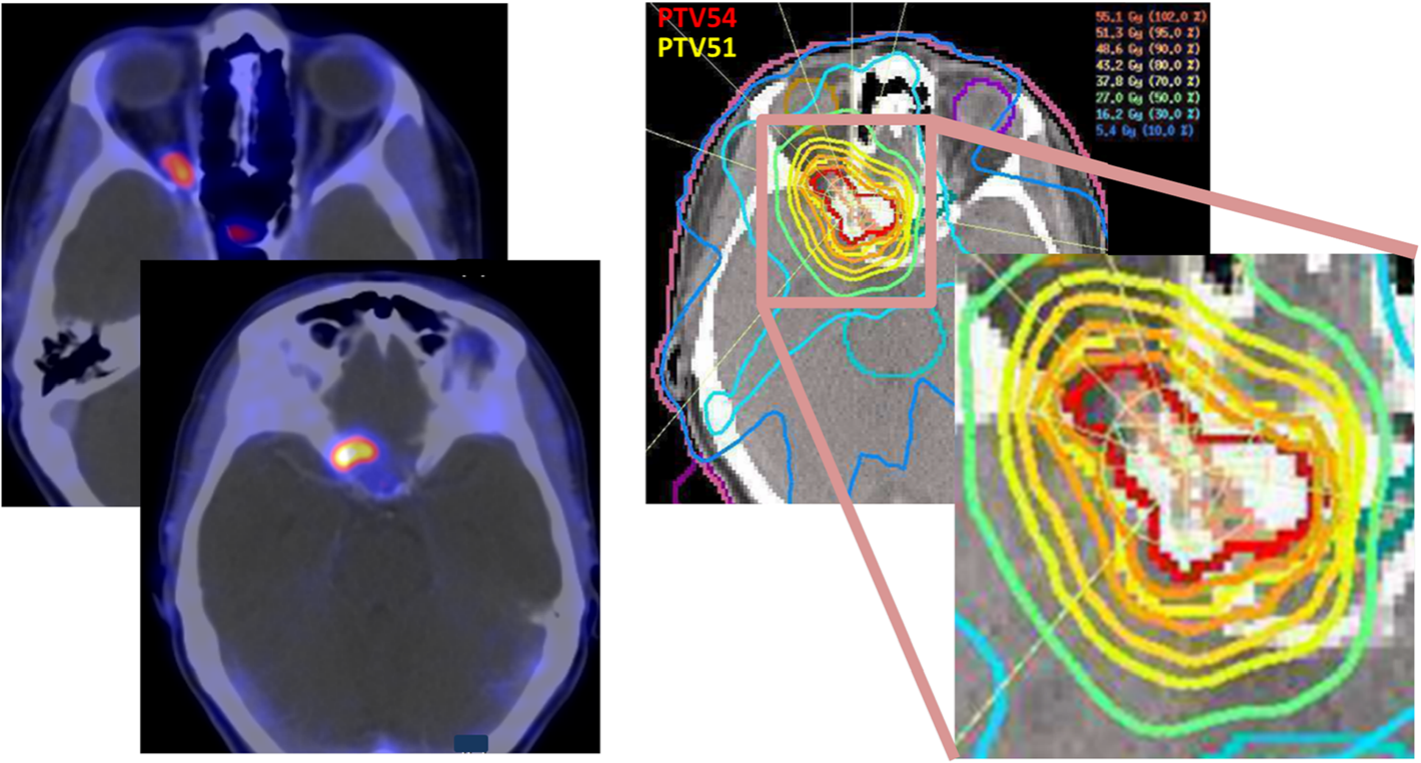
Typical example of a somatostatin-receptor-analogon PET-CT (68-Gallium-DOTATATE) with an average standard-uptake-value (SUV) of 4.8. Physiological uptake of the hypophysis can be seen in the upper image. The radiation plan shows steep dose gradients with sparing of the contralateral optic nerve
